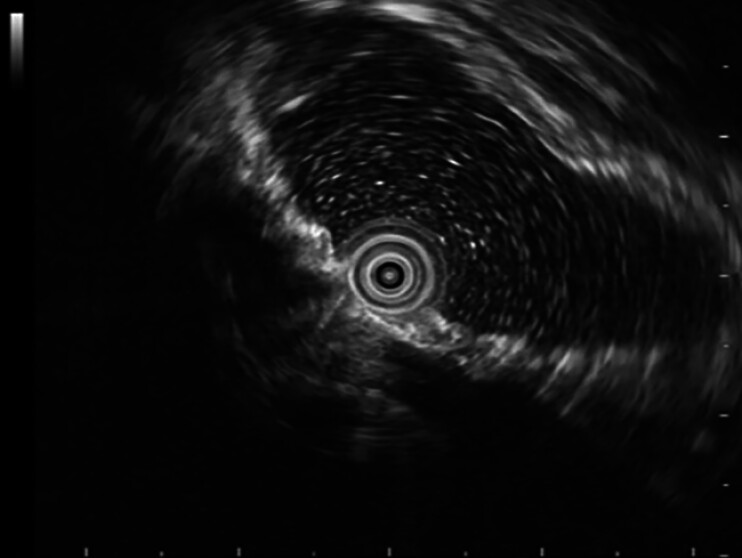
The ultrasound colonoscopy (20 MHz) revealed penetration of the entire rectal wall by the intrauterine device (IUD).
